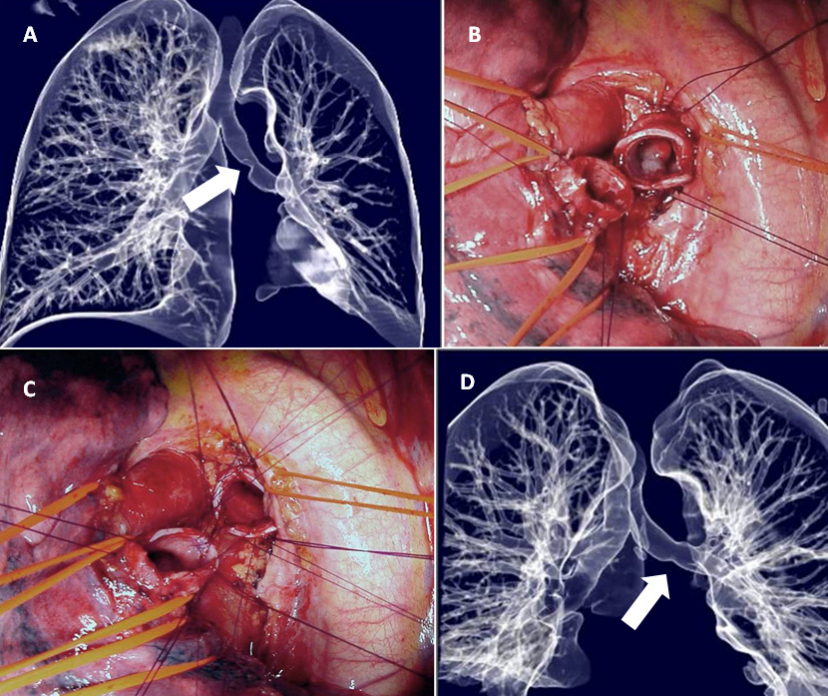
Typical carcinoid. A: Preoperative airway volume rendering (CT) of bronchial tree. B: Surgical field after distal left main bronchus interruption (the polipoid tumor growth is visible within the proximal stump). C: Surgical field after bronchial resection. Construction of end-to-end anastomosis according to Grillo. D: Postoperative CT rendering.
